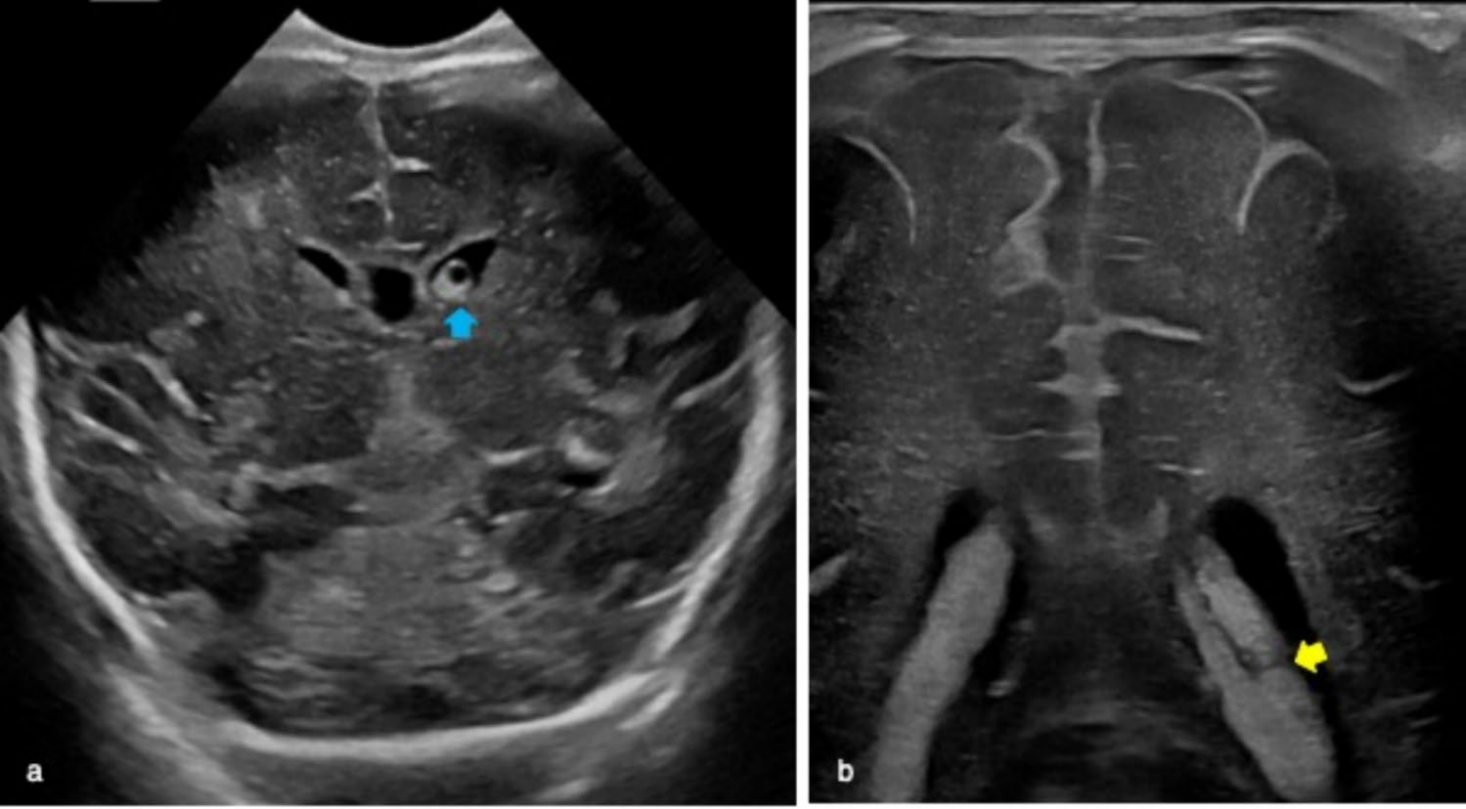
Coronal planes of cerebral ultrasound performed on premature infant demonstrated left well-defined cyst (blue arrow in a) and hyperechoic central cleft defining split choroid sign (yellow arrow in b). These are typical findings of choroid plexus cyst
